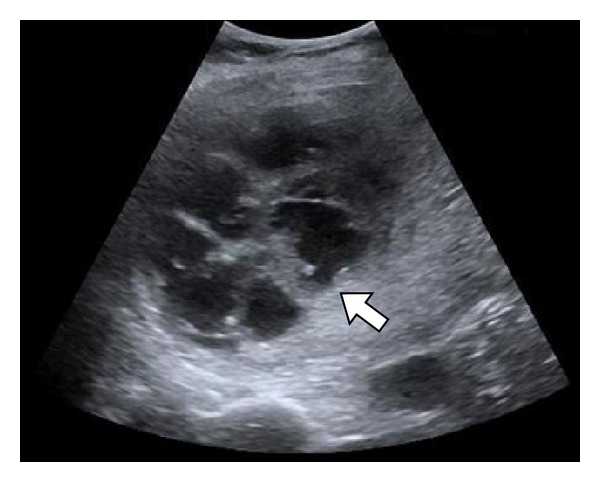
Right upper quadrant ultrasound showing a complex hepatic lesion with multiple internal septations (arrow).
